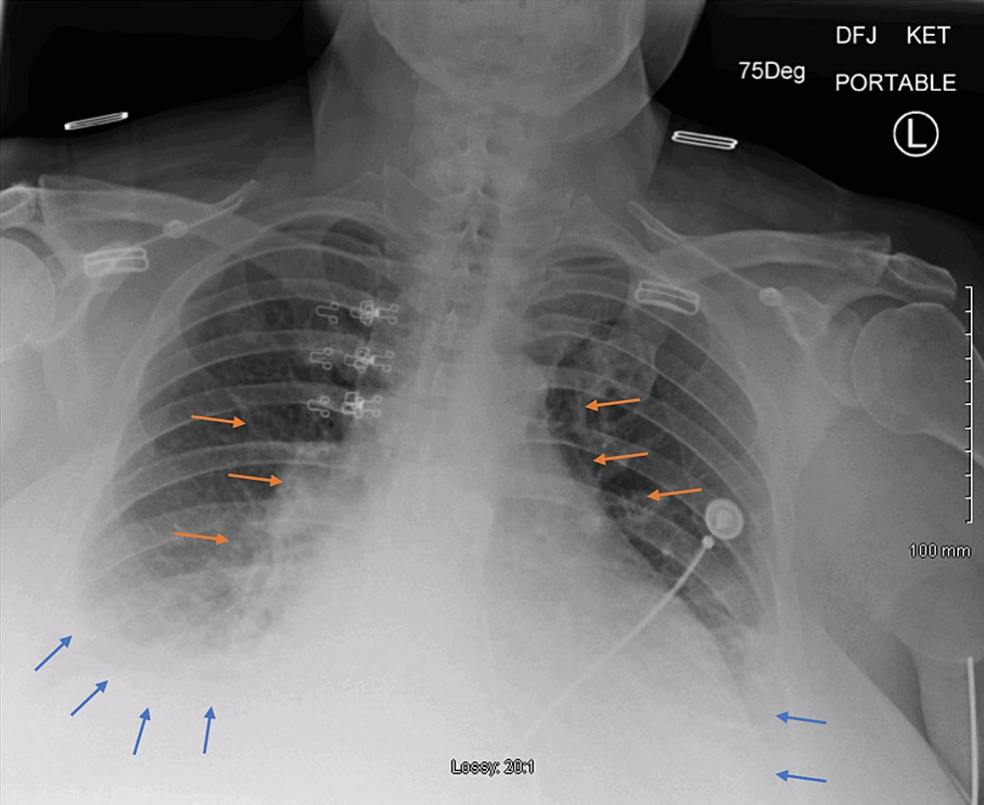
Chest x-ray showing mild cardiomegaly with interval development of bilateral pleural effusions (blue arrows) with bibasilar atelectasis, pulmonary vascular congestion, and edema (red arrows).
